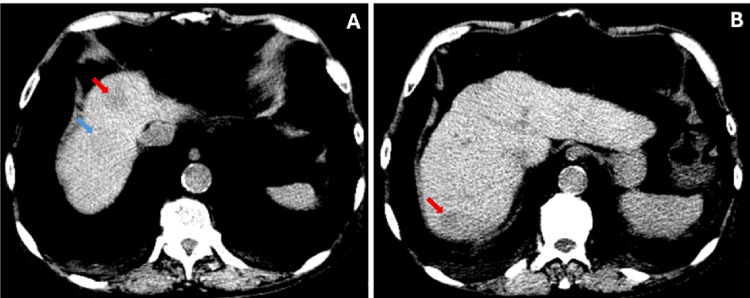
Abdominal CT scan without contrast in November 2025 revealed multiple hypodense hepatic nodules, with the largest ones in (A) segments IVa and VIII (red arrow and blue arrow, respectively) and in (B) segment VII, which were not present in the MRI performed in January 2024.
